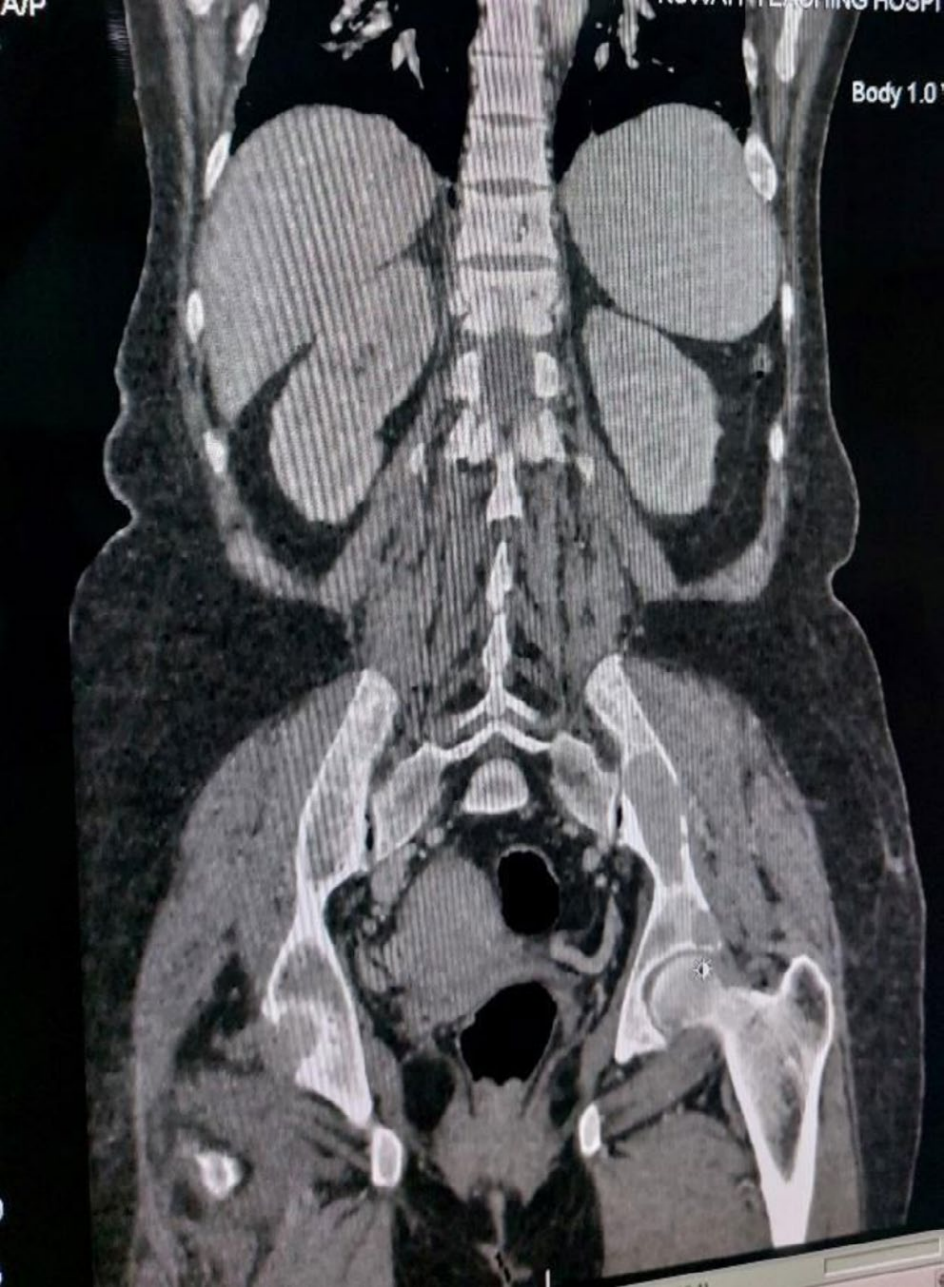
Coronal view of CT abdomen and pelvis showing hypodense lesions in the left iliac blade.
